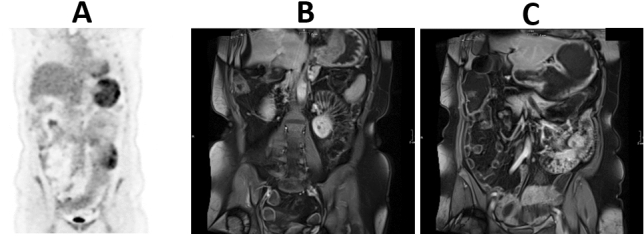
Pre-operative FDG-PET imaging shows an increased metabolic activity of the stomach with a radiotracer avid area (asterisk) in the larger curvature (SUV max 16.9). Hypermetabolic activity is also present in a small bowel loop in the left hypocondrium (arrowhead) (A). Magnetic resonance enterography shows increased enhancement of the gastric wall (B) and the first jejunal loop presents thickened walls and a hypervascularized mesentery with reactive lymph nodes (C)
